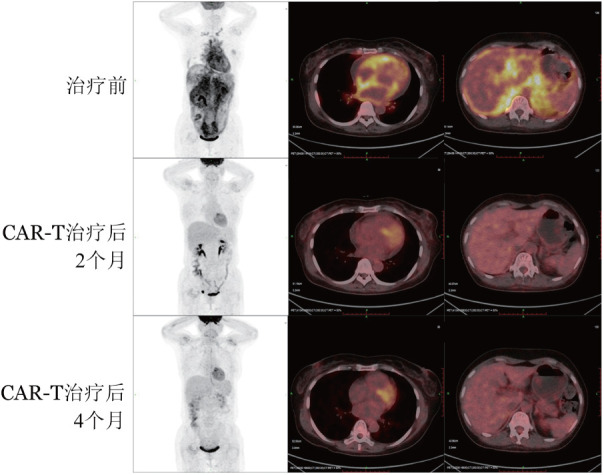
PET-CT示患者（例6）CD19 CAR-T细胞治疗后骨髓外复发病灶缓解 CAR-T细胞：嵌合抗原受体T细胞
